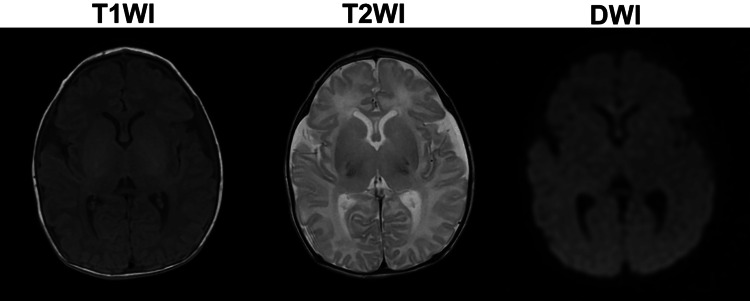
Magnetic Resonance Imaging (MRI) of the Neonatal Brain. MRI of the neonatal brain was performed on the 19th day of life and revealed no evidence of hypoxic-ischemic encephalopathy. T1WI, T1-weighted image; T2WI, T2-weighted image; DWI, diffusion-weighted image
